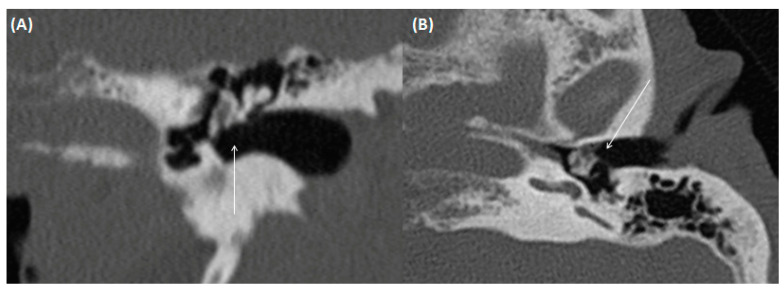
CT of a 73-year-old female patient with a history of chronic mastoiditis. Note the thickening of the eardrum and the adjacent ligaments of the ossicular chain as a sign of tympanosclerosis in coronar (A) and transversal (B) figure.
